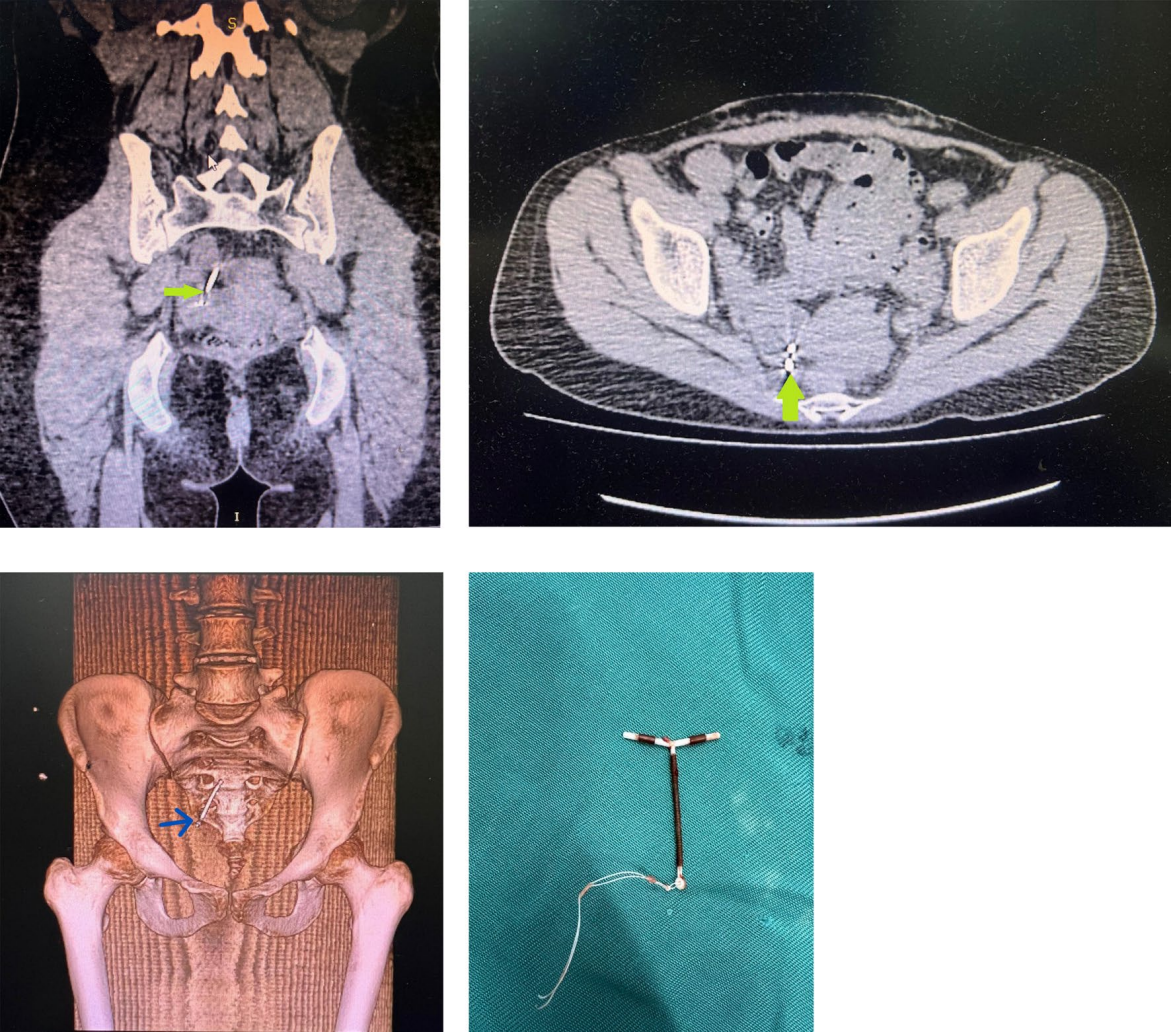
(a) CT—Pelvis coronal displaying a transmigrated T‐shaped IUD with long arm directed superomedially and short T‐arm potentially impinging onto a right ovarian surface. (b) CT—Pelvis axial view showing IUD in close proximity to right ovary. (c) CT 3D view of the IUD. (d) Showing the actual translocated IUD postlaparoscopic retrieval.
